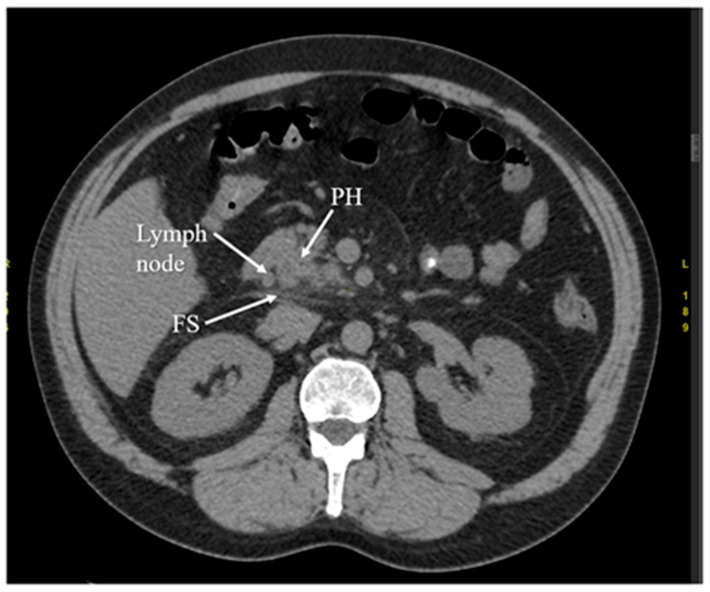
Early phase of the acute pancreatitis with stranding of surrounding fat (FS) and single, enlarged lymph node. The arterial phase of CT. PH—head of the pancreas.
